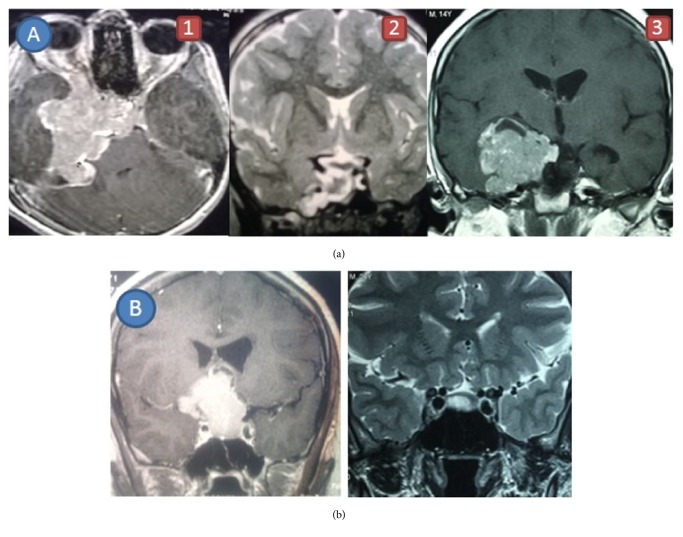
(a) MRI images of Case 1 at diagnosis (1), initial response to dopamine agonist (2), and recurrence of dopamine agonist resistant tumour (3); (b) MRI images of Case2 at diagnosis and showing tumour shrinkage to subcentimeter level after 5 years of dopamine agonist therapy.
